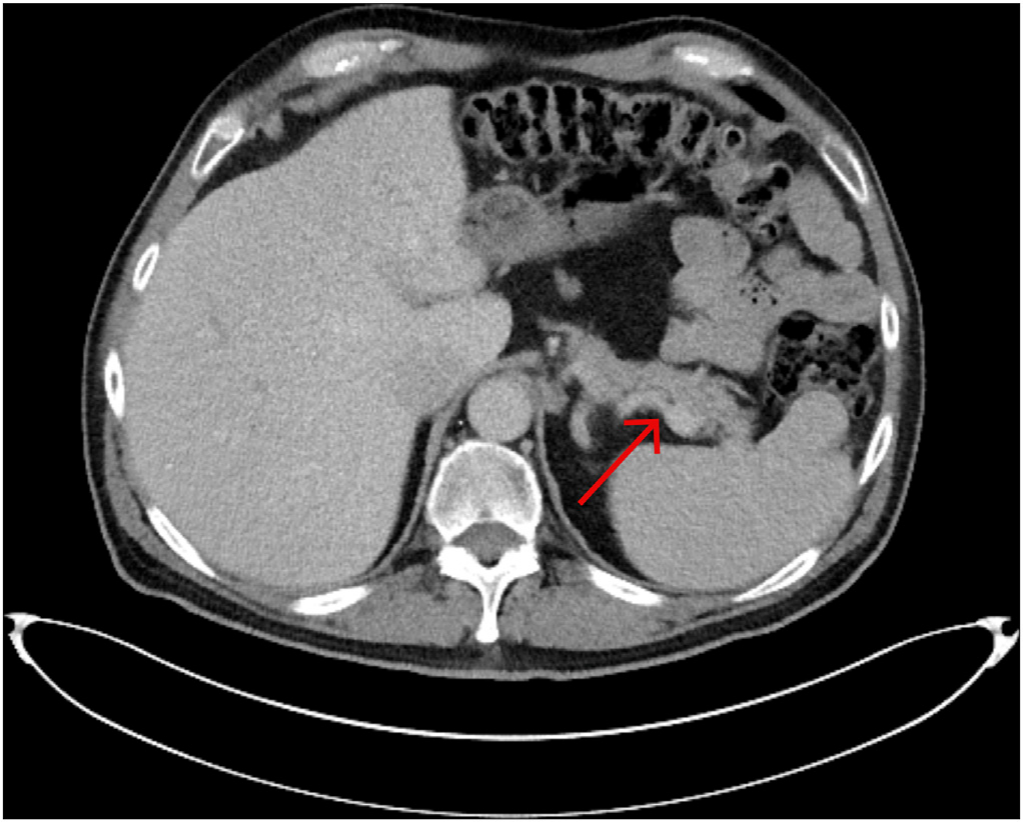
CT abdomen with intravenous contrast, transverse plane, the pancreas (red arrow) appears structurally normal without signs of inflammation or masses.
